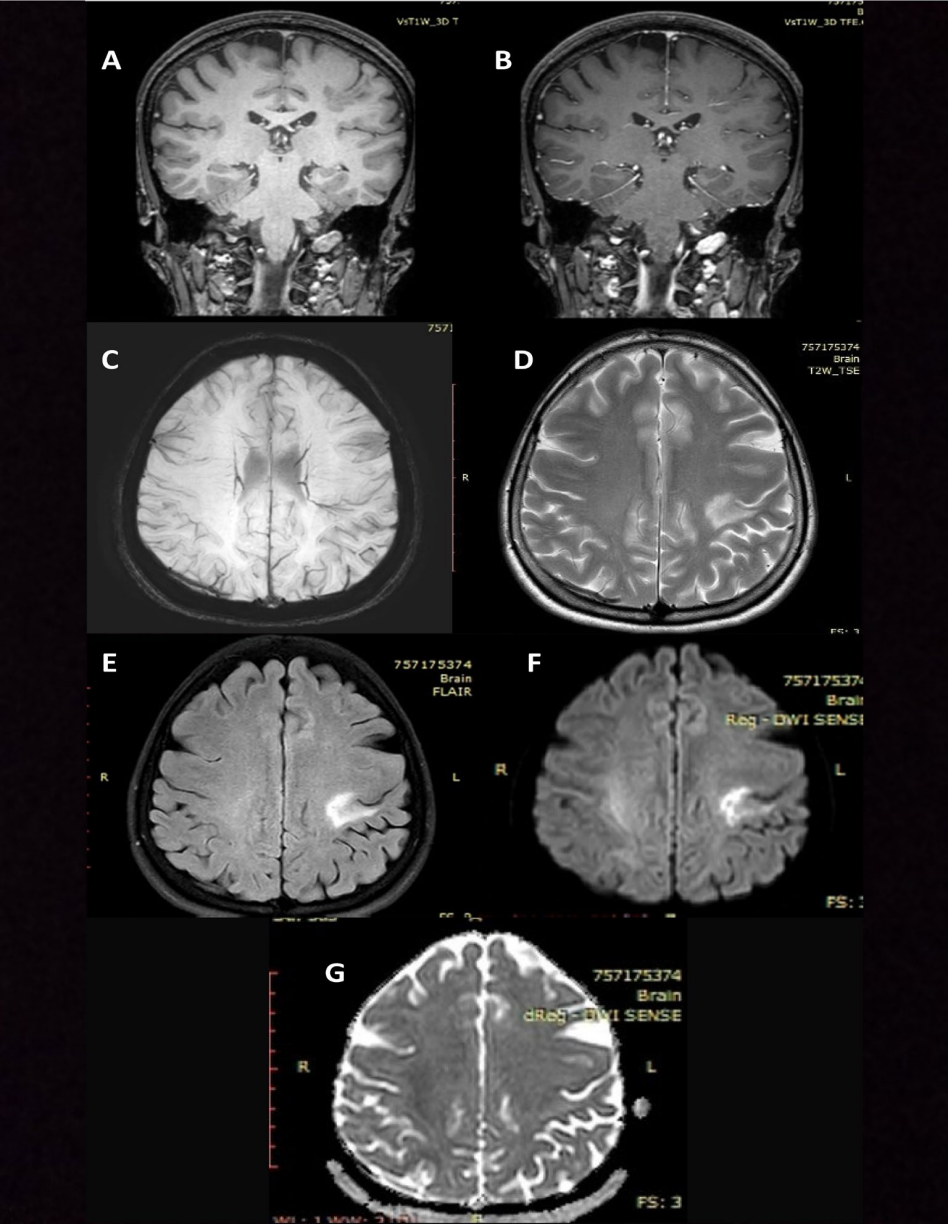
Third Brain MRI done after 5 months from first presentation. (A) T1 Coronal Pre Contrast, (B) T1 Coronal Post Contrast, (C) SWI, (D) T2 Axial, (E) FLAIR Axial, (F) DWI, (G) ADC. This magnetic resonance imaging (MRI) shows a residual area of T2w/flair white matter high signal intensity area of 16 × 15 mm in size, no enhancement and no diffusion restriction were seen, with prominent central vein sign as seen on susceptibility weighted imaging (SWI).
